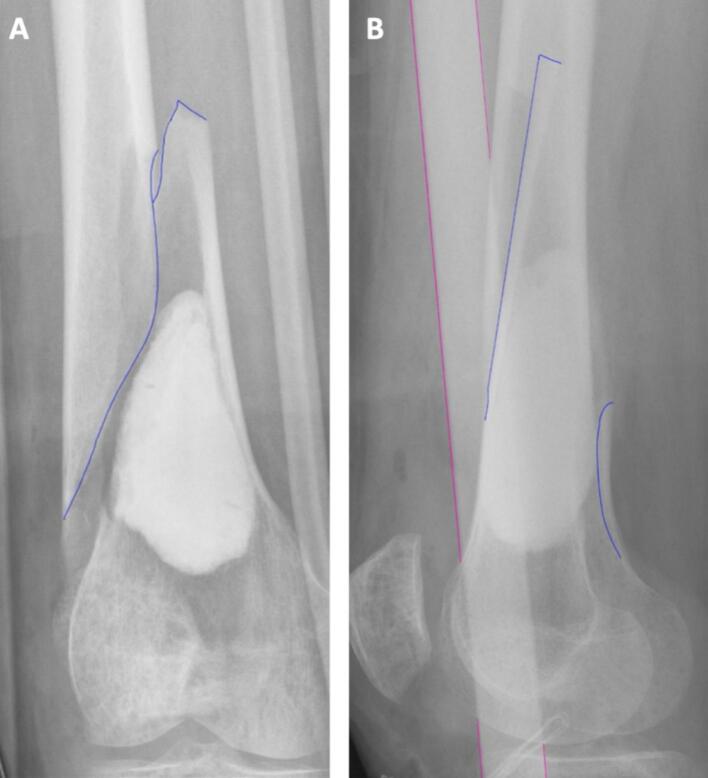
Anterior posterior (AP) (A) a lateral X-ray images (B) of a fracture (blue line) after curettage of an ACT with cement void filling. The pink line indicates the splint. (For interpretation of the references to colour in this figure legend, the reader is referred to the web version of this article.). (For interpretation of the references to colour in this figure legend, the reader is referred to the web version of this article.)
